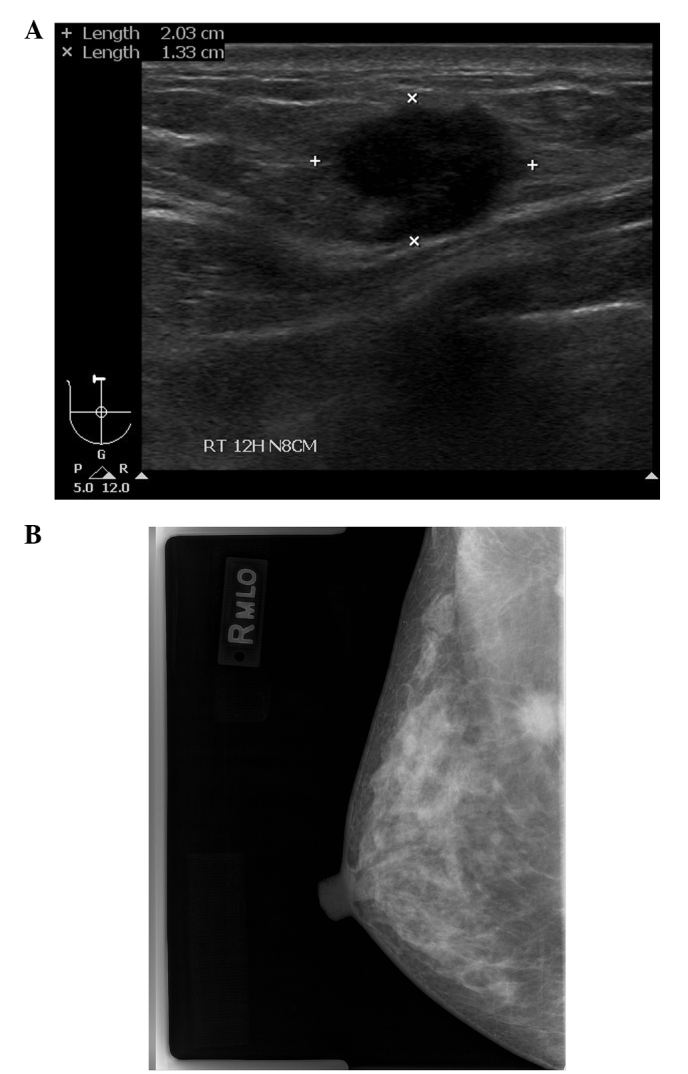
Radiological findings of the right breast. (A) Mammography showing a spiculate hyperdense lesion in the upper portion of the right breast. (B) Ultrasonography (USG) scan showing an irregularly-shaped hypoechoic lesion in the right breast.
